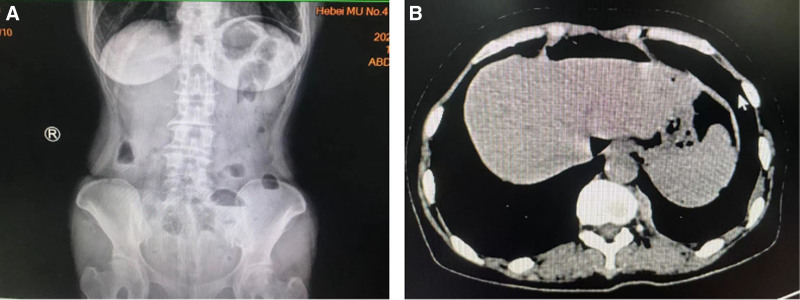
(A) Standing abdominal plain film. (B) Abdominal CT. CT = computed tomography.
